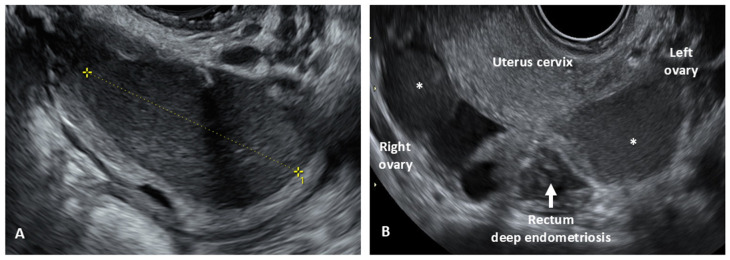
Transvaginal ultrasound (TVS) appearance of ovarian endometrioma. (A) A unilocular cystic lesion with smooth walls and a “ground glass” appearance is the most typical presentation of an endometrioma; (B) bilateral endometriomas (with asterisks) giving the appearance of “kissing ovaries”.
